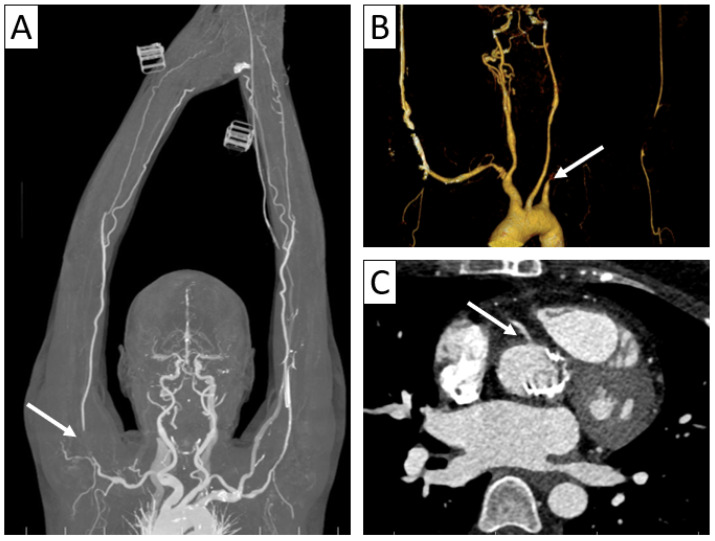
Complications of vasculitis—stenoses and occlusions. (A) Maximum intensity projection (MIP) of 3D-CTA showed brachial sub-occlusion (arrow) in a patient with parietal thickening of up to 4 mm in the supra-aortic trunks with extension to the axillary and brachial arteries. (B) Supra-aortic trunk CTA 3D volume rendering in a 38-year-old patient with TAK and Behcet’s disease overlap showed occlusion of the left subclavian and axillary arteries (arrow) with weak brachial artery distal reperfusion. (C) Axial cardiac CT in a 35-year-old patient with TAK revealing aortic wall thickening extending to the right coronary artery ostium with minor stenosis (arrow).
